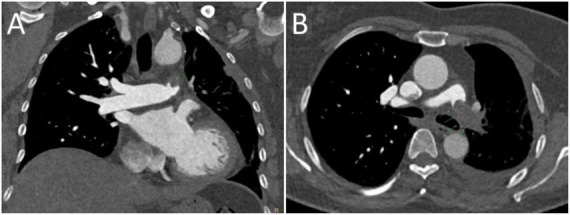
Computed tomography pulmonary angiography (CTPA) of the left pulmonary artery. (A) Coronal view. (B) Axial view. Green arrows indicate a low-attenuation intraluminal filling defect within the left main pulmonary artery.
